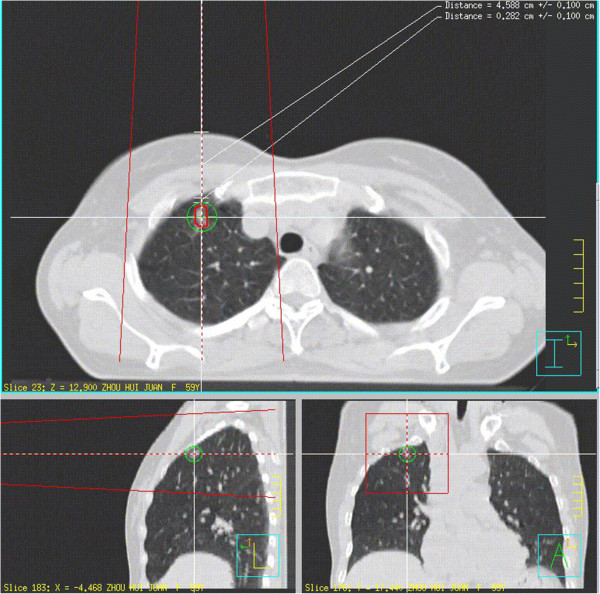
Image generated using radiation therapy planning system based on images and data collected from previous CT scan.
